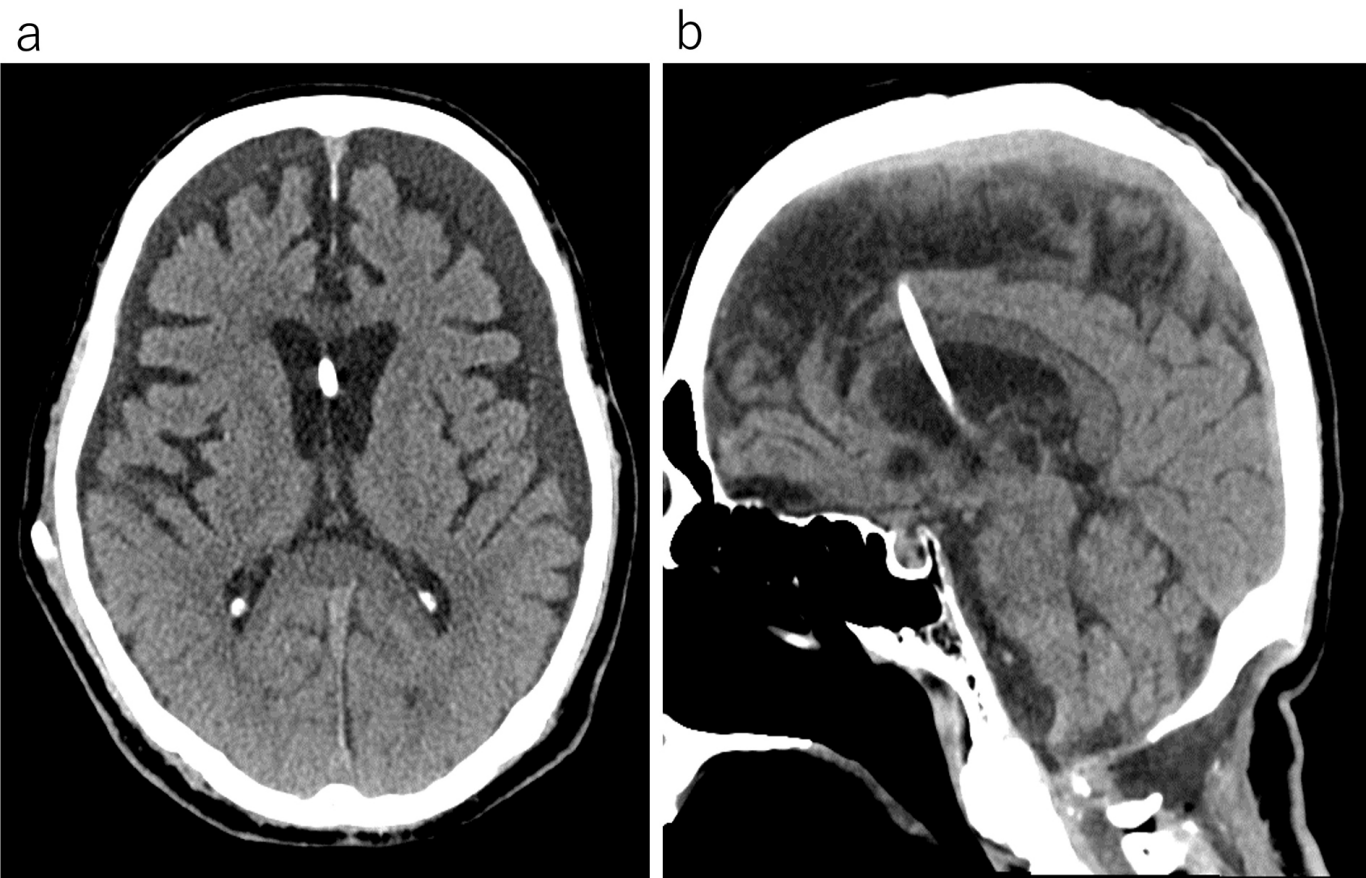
This computed tomography scan of the head, performed after ventriculoperitoneal shunt surgery, shows that the ventricles have returned to normal size (a) and the pseudomeningocele has significantly decreased (b).
